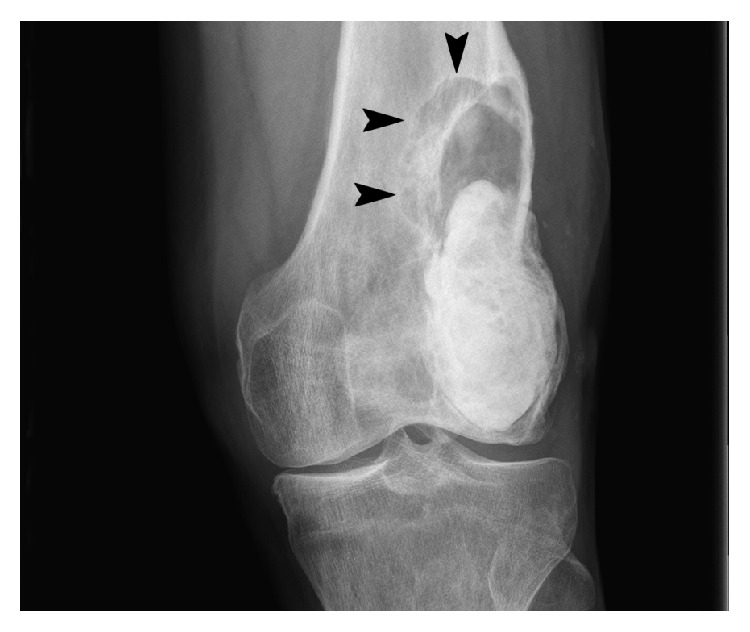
Frontal left knee radiograph (July 2014) demonstrated increased well-defined lytic focus (arrows) surrounding the radiopaque PMMA, suggestive of malignant transformation.
